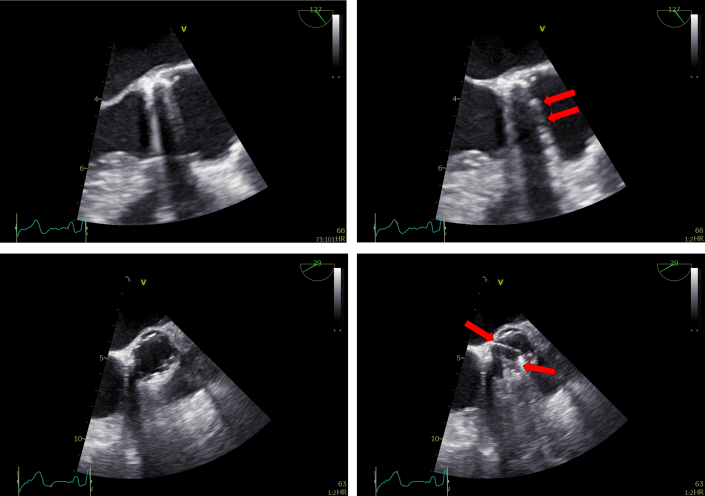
Transesophageal Echocardiography High-esophageal long-axis views (upper panels) and short-axis views (lower panels) are displayed in diastole (left panels) and systole (right panels). The red arrows highlight symmetric disk motion. Leaflet mobility is preserved, with no paravalvular leak or other evidence of prosthetic valve dysfunction.
